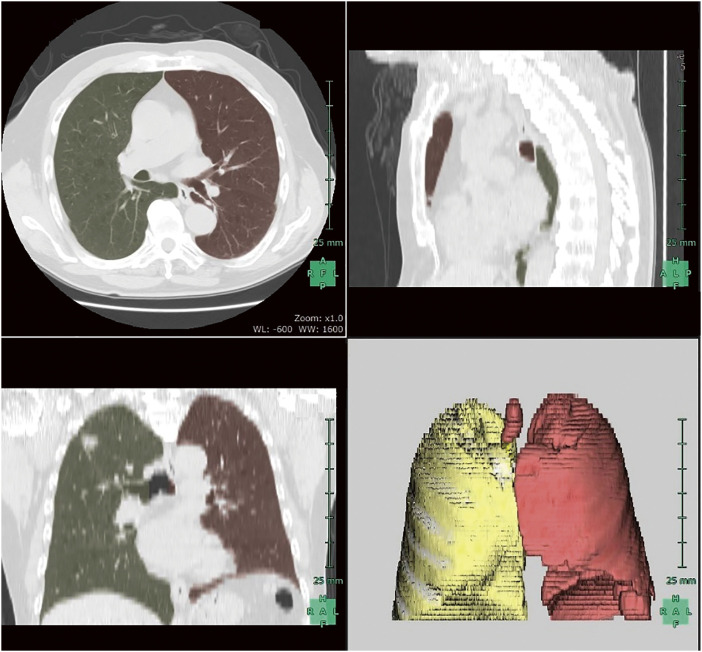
Lung volume assessment using SYNAPSE VINCENT (Fujifilm Co.). Lung fields were automatically identified based on computed tomography images, and 3-dimensional reconstructions were generated. Following manual verification, total and left lung volumes were calculated.
